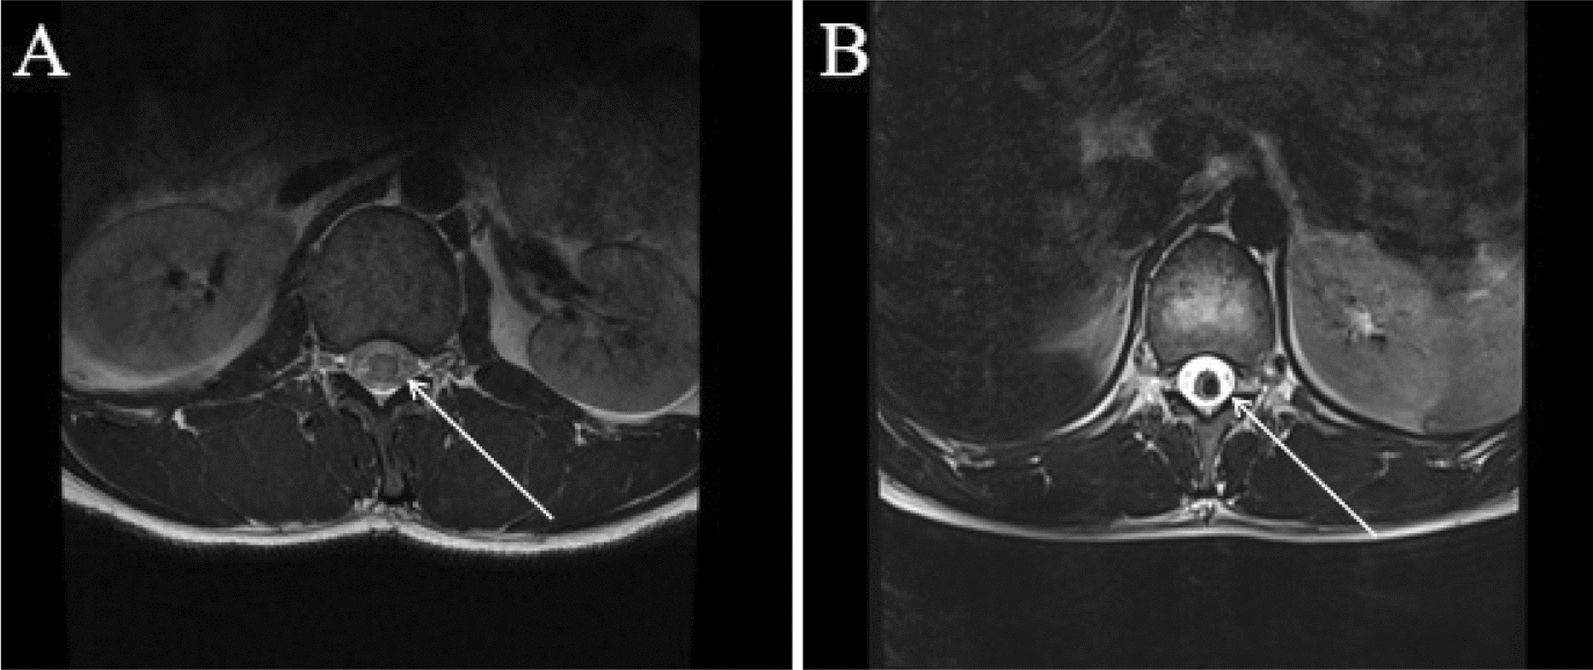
Axial T2 weighted magnetic resonance image (A the 2th day of admission and B the 10th day of admission) of the lumbar spine with spindle-shaped hematoma sign. Signal of the spinal cord can be seen along the the fiber tracts (arrows)
